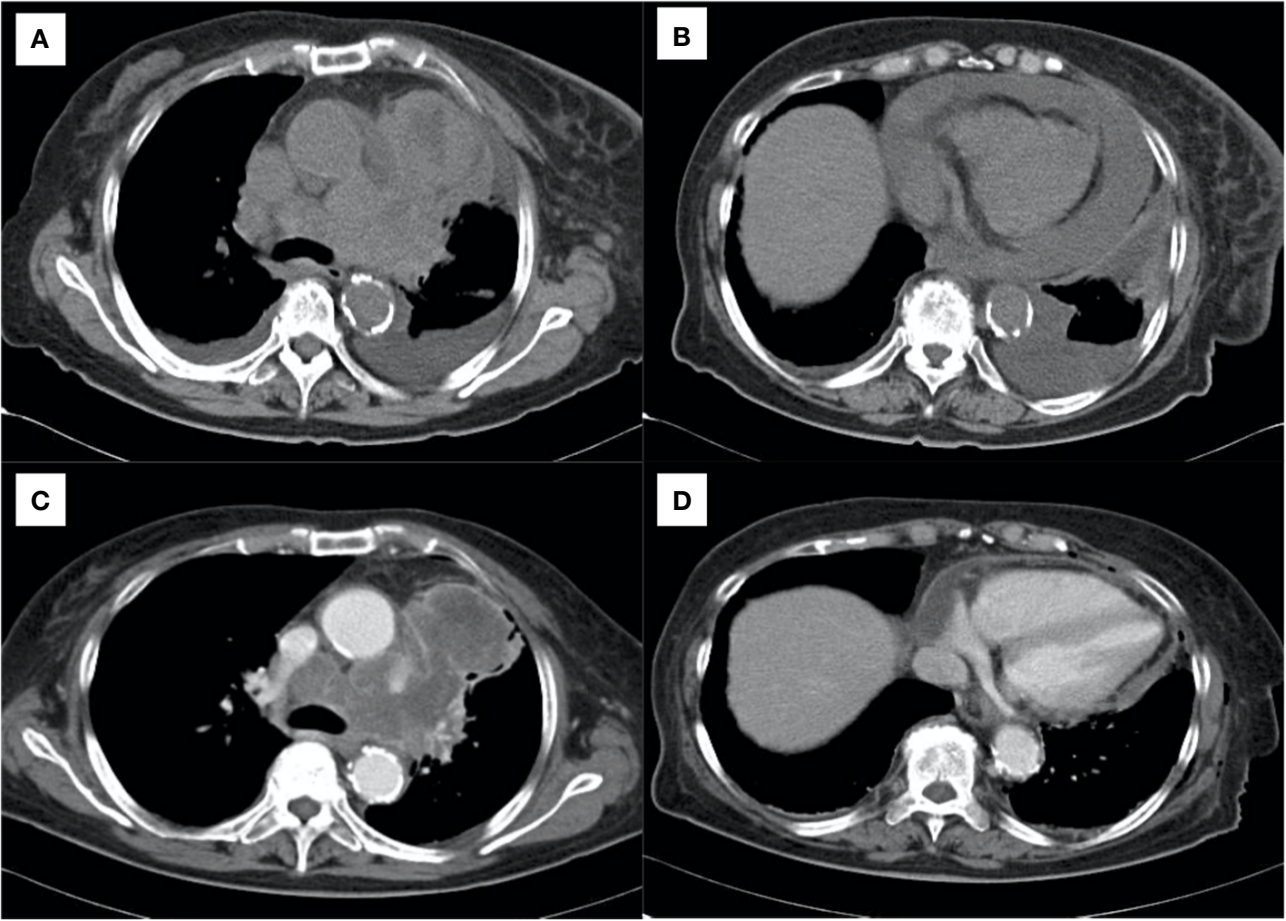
Chest computed tomography showing (A) primary lung cancer and bilateral pleural effusions and (B) pericardial effusion. (C, D) After drainage of the effusions and pleurodesis, showing control of the effusions before starting systemic chemotherapy.
